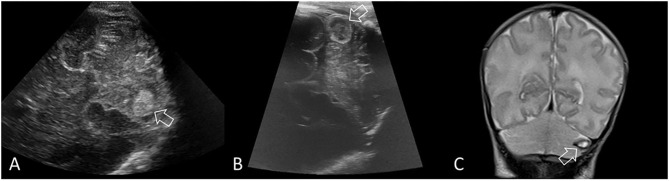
Limited cerebellar hemorrhage. Ultrasound scan in a preterm infant (GA 29 weeks) with a limited CBH (>4 mm but <1/3 of the cerebellar hemisphere). (A) Coronal scan through the right mastoid fontanel 5 days after birth shows a rounded echogenic lesion in the left cerebellar hemisphere (arrow), indicating intraparenchymal hemorrhage. The fourth ventricle is borderline dilated (not shown). (B) Repeat coronal scan through the left mastoid fontanel at 2 weeks after birth shows expected evolution of the hemorrhage (arrow), which is becoming echolucent, indicating early cystic degeneration. (C) Coronal T2-weighted MRI at 6 weeks after birth shows continued evolution of the hemorrhage with smaller cystic cavity that displays uniform central T2 hyperintense signal and thin hypointense rim (arrow, hemosiderin and ferritin). Surrounding cerebellar edema has resolved with local destruction and atrophy of that hemisphere. The appearance of high intensity on T1-weighted (not shown) and T2-weighted inside the hemosiderin rim stages the hemorrhage to the late subacute phase (extracellular methemoglobin).
